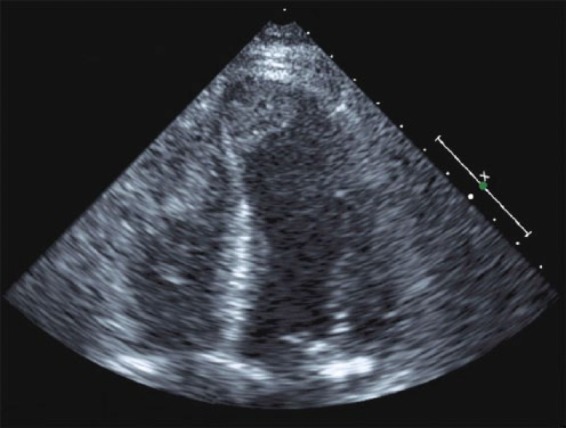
Two-dimensional transthoracic echocardiogram demonstrating large biventricular mural thrombi.
